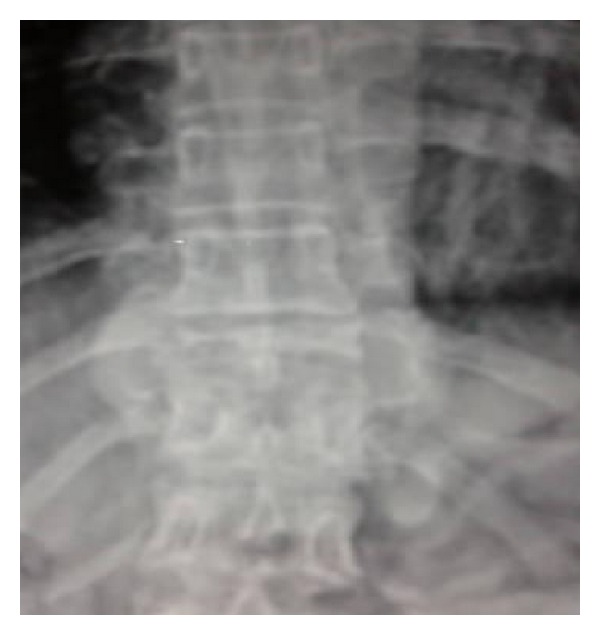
X-ray dorsolumbar spine showing vertebra plana of T10 vertebra. Disc space is well maintained.
